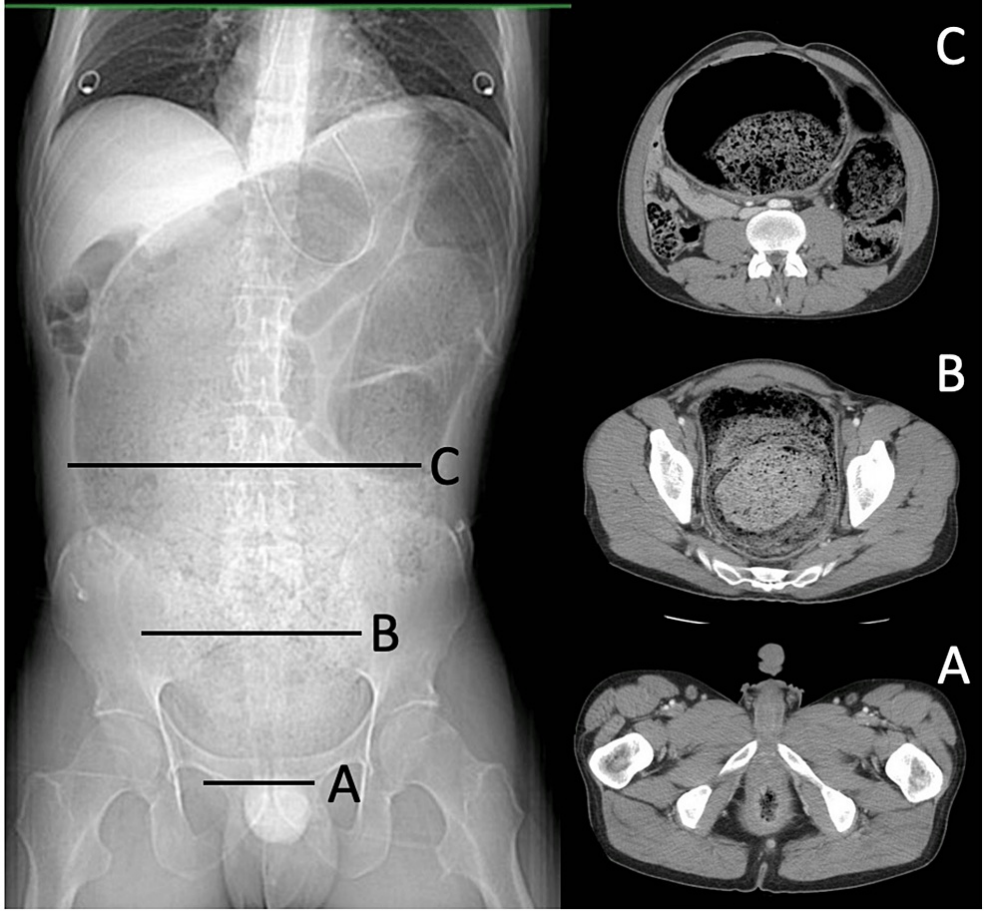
CT scan of the abdomen/pelvis with contrast. A. Anus. B. Transition point: dilated rectum with stool; bladder compressed right and anteriorly. C. Redundant L colon/R colon with obstruction. CT: computed tomography; L: left; R: right
